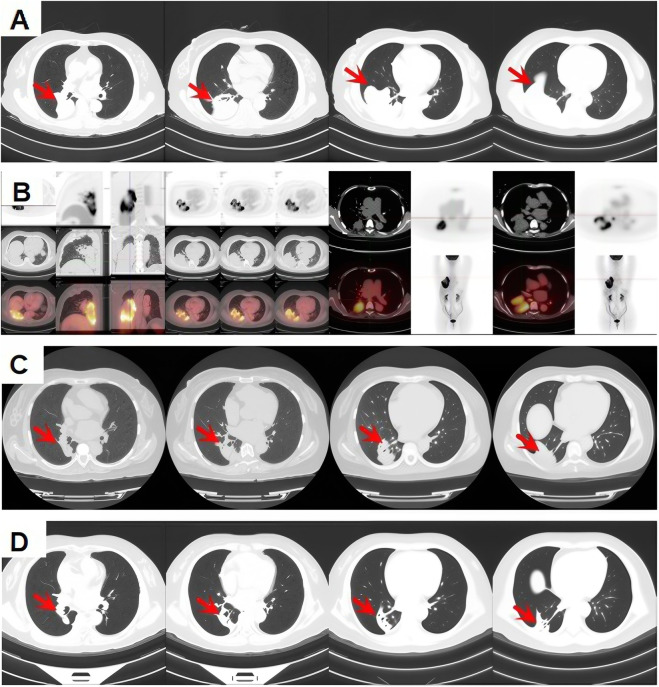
Radiologic Assessments Before and After Neoadjuvant Alectinib. (A) Baseline contrast-enhanced chest CT at diagnosis shows a large lesion (8.3 cm) in the right lower lobe (arrows). (B) Baseline positron emission tomography-computed tomography (PET-CT) image demonstrates intense FDG avidity in the primary tumor (SUVmax 17.59) and station 11R/7 lymph nodes (SUVmax 4.99). (C) CT scan after 4 weeks of alectinib treatment shows a marked partial response, with significant regression of both the primary tumor (arrows) and nodal disease. (D) CT scan after 8 weeks of alectinib therapy demonstrates continued tumor regression (arrows) and consolidation of the treatment response.
